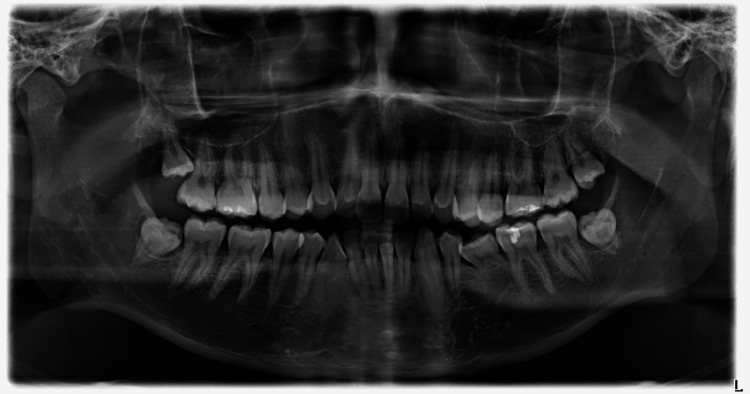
Panoramic x-ray of the patient, where the six maxillary anterior talon cusps appear clearly. The retained primary left second molar, tooth #75, appears to have partially resorbed roots, due to the congenitally missing tooth #35. As there are no clinical signs of mobility, the tooth may be retained for an unknown time in the mouth.
